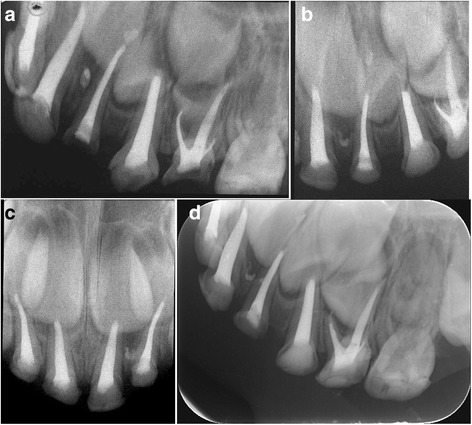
Twenty-four months follow-up of MPRCF. a Radiograph of 52–64 treated with MPRCF taken 6 months postoperatively. b, c Radiograph taken 18 months postoperatively showing partly resorption of overfilled materials in 62. d Radiograph taken 24 months postoperatively showing intact filling
